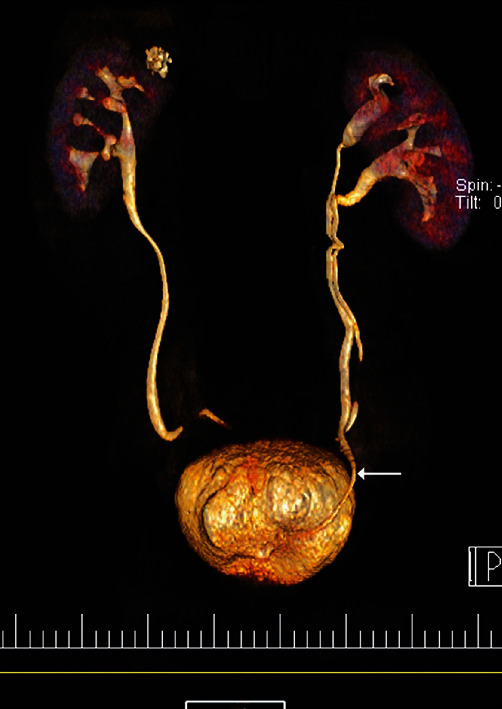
CT urogram showing duplex (i.e., with double ureters) left kidney. The left upper moiety ureter that empties into the bladder at an ectopic site is indicated by the arrow.
